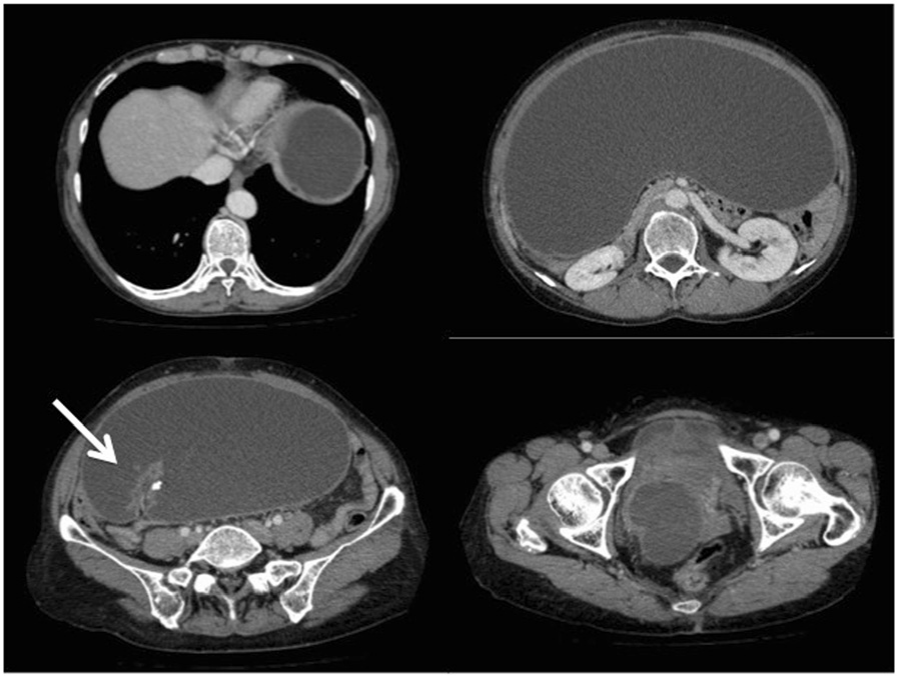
Contrast-enhanced computed tomography showed a well-encapsulated, unilocular cystic mass which occupied the entire abdomen from just below the diaphragm to the pelvis. *Arrow* showed a dendritic structure protruding into the tumor cavity
